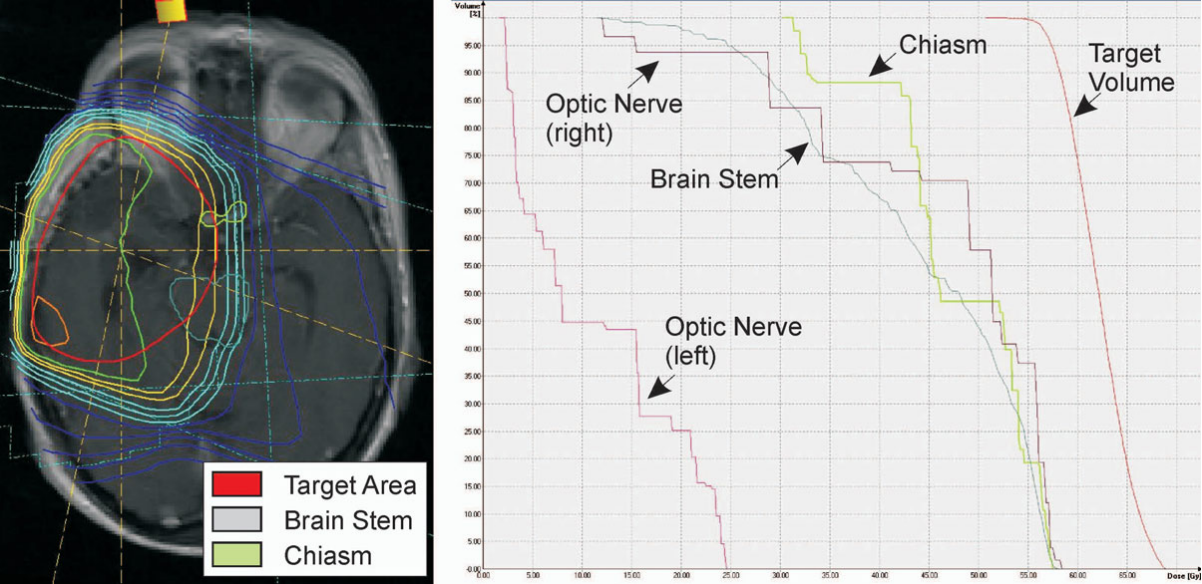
Target volume and dose-volume histogram. Figure 2A Magnetic resonance imaging after open surgery with isodose lines from 10 to 110%. Target volume is marked in red, chiasm in green and brainstem in blue. Figure 2B Dose-volume histogram (DVH) of the radiation shows the dose given to the percentage of OAR (organ at risk) volume, i.e. less than 20% of the chiasm was irradiated with 56 Gy within 6 weeks.
